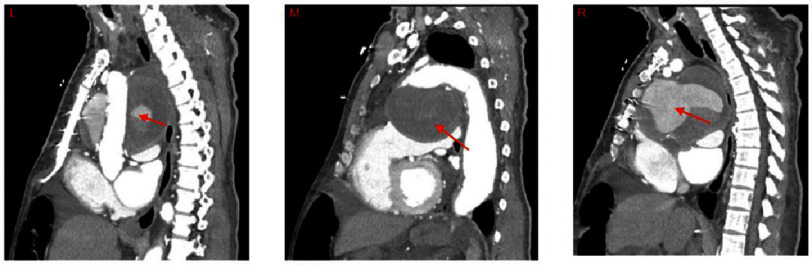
Sagittal Images of CT scan of a patient on VV ECMO with an aortic pseudoaneurysm at the distal suture line of a prior aortic arch replacement. Left: Large pseudoaneurysm (arrow) behind the aortic graft, compressing the trachea and esophagus posteriorly. Middle: Large pseudoaneurysm below arch (arrow), compressing heart inferiorly. Right: Large pseudoaneurysm (arrow) just posterior to the sternum, compressing the heart inferiorly while compressing the esophagus and trachea posteriorly. Abbreviations: CT - computed tomography, CTA - computed tomography angiogram, VV ECMO - venovenous extracorporeal membrane oxygenation.
